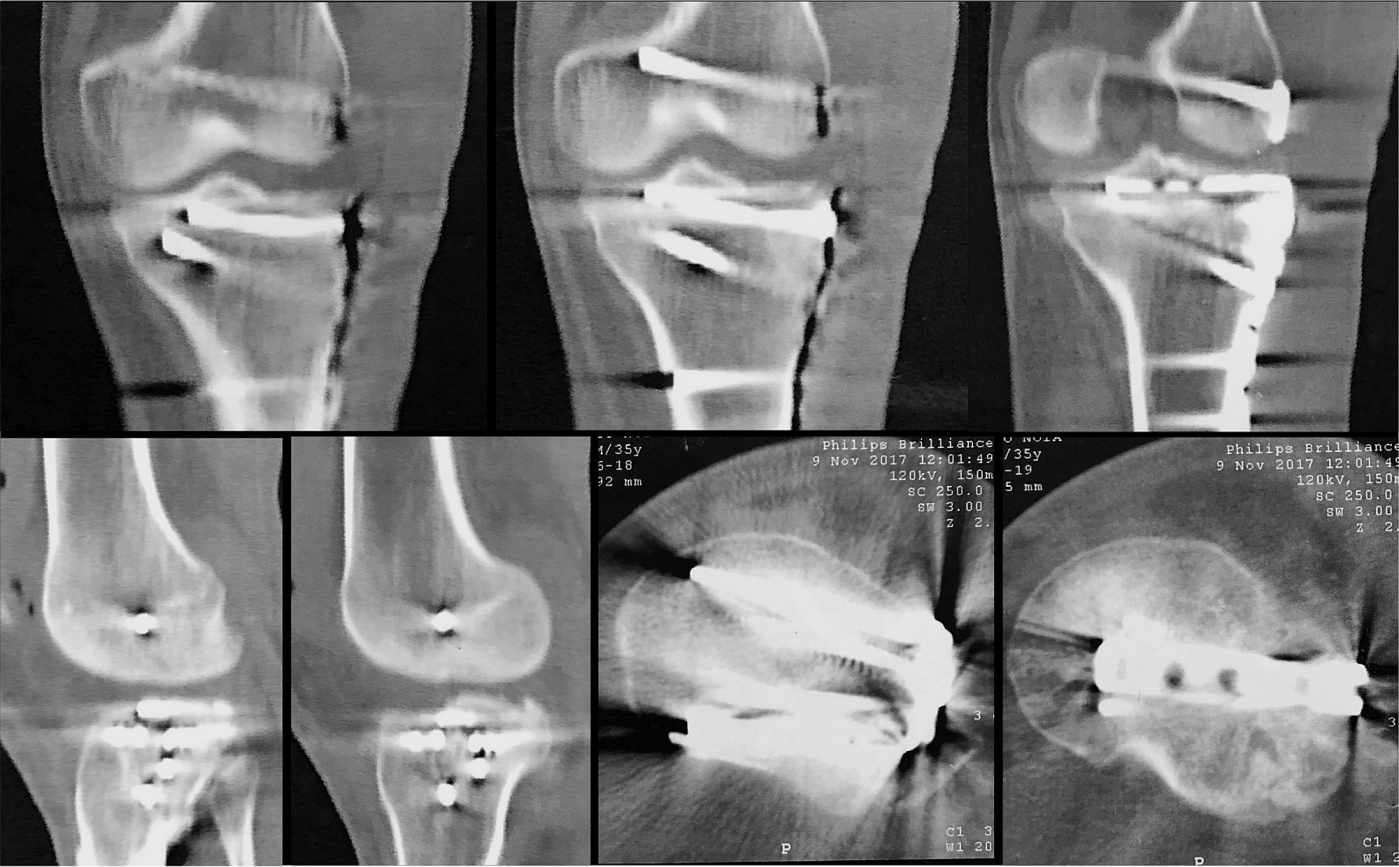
GM, 35-year-old, male patient, fall from a motorcycle Immediate postoperative CT scan of the left knee after the second operation at day 4 – anatomic reduction was obtained CT: computed tomography
